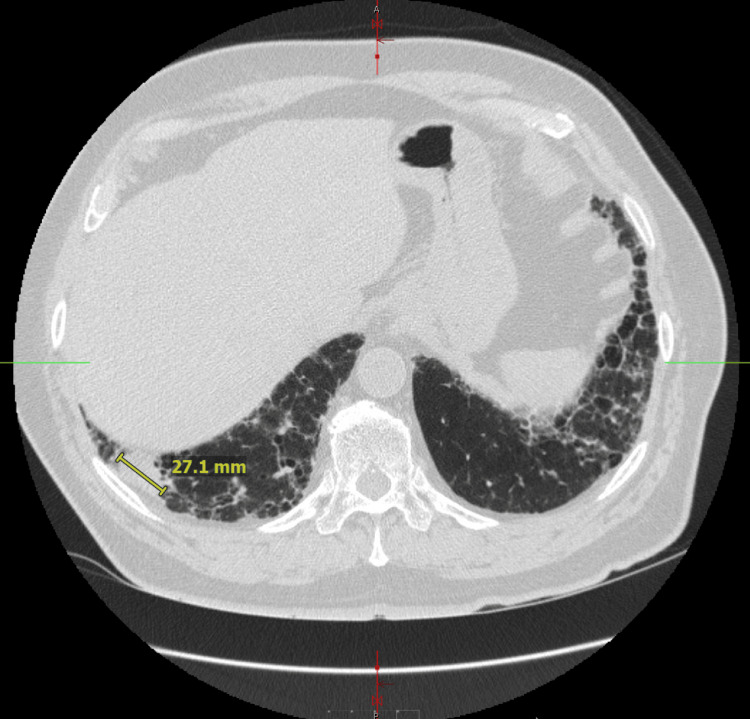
High-resolution CT Chest: 2.7 cm solid nodule in the posterior basal right lower lobe
